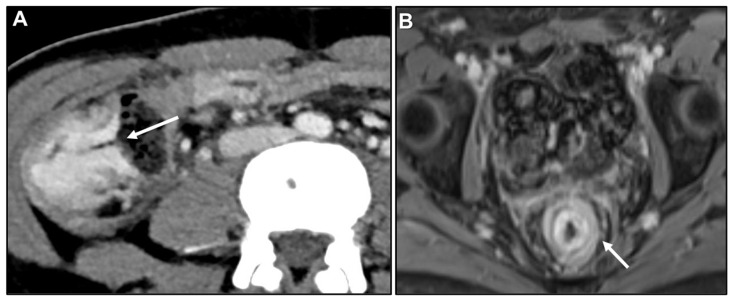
Malignant target sign. (A) Axial contrast-enhanced CT. Linitis plastica-like metastasis to the caecum from invasive lobular carcinoma (arrow). (B) MR image: Axial CE-GRE-T1WI. Linitis plastica-like rectal metastasis from breast adenocarcinoma (arrow).
